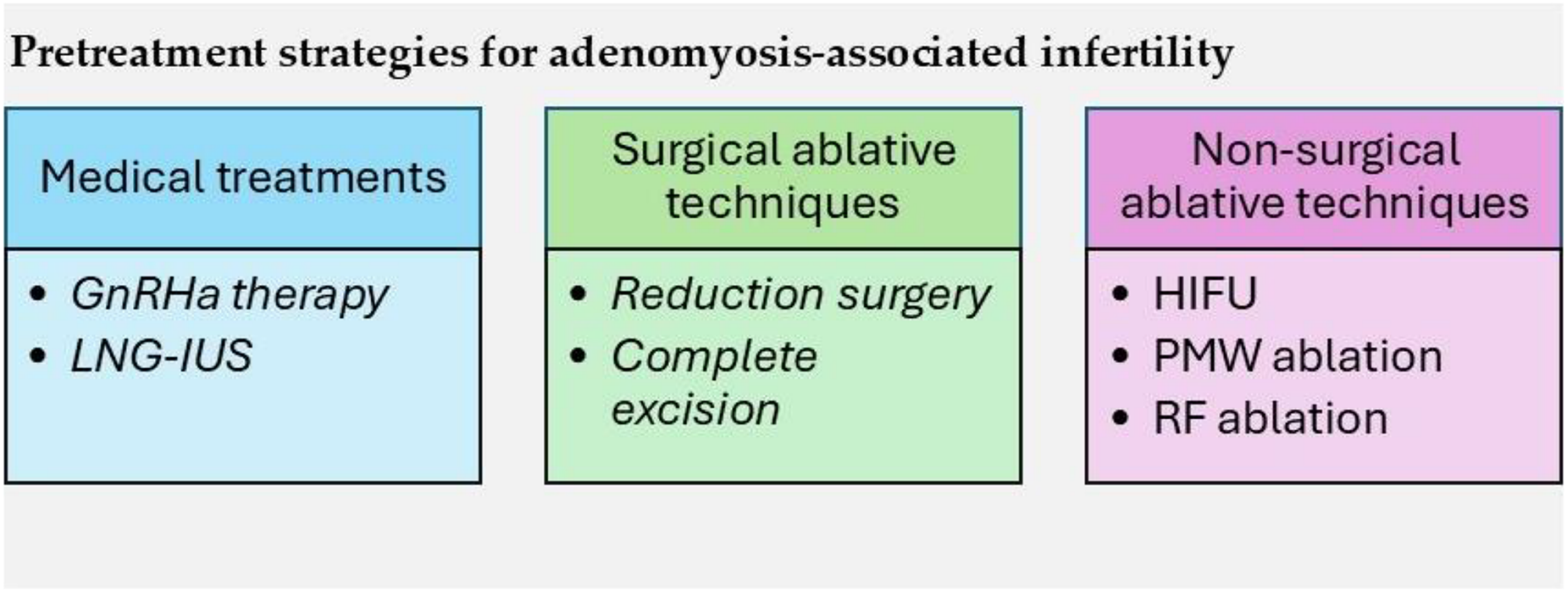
Pretreatment strategies for adenomyosis-associated infertility. GnRH, gonadotropin-releasing hormone agonist; LNG-IUS, levonorgestrel intrauterine system; HIFU, high-intensity focused ultrasound; PMW, percutaneous microwave; RF, radiofrequency.
